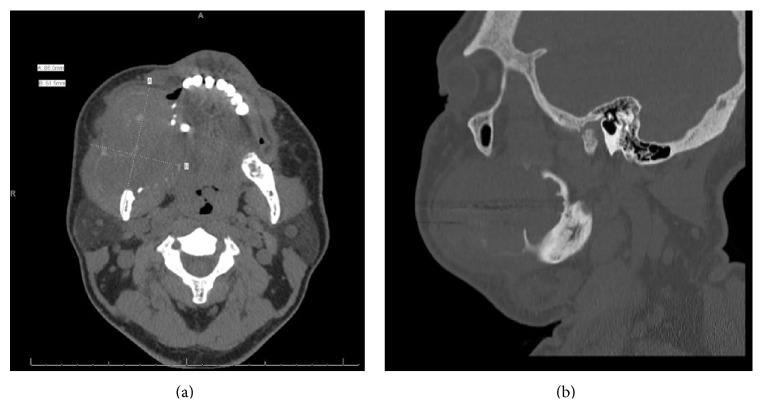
(a) Axial CT image. (b) CT scan, sagittal view.
